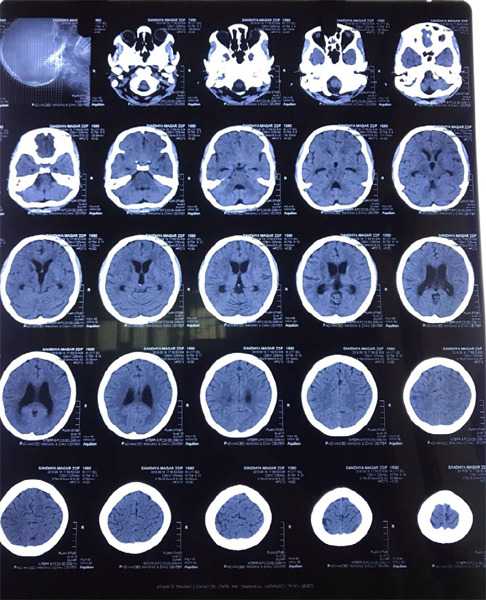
Axial plain CT scan of head showing infarct and communicating hydrocephalus.
